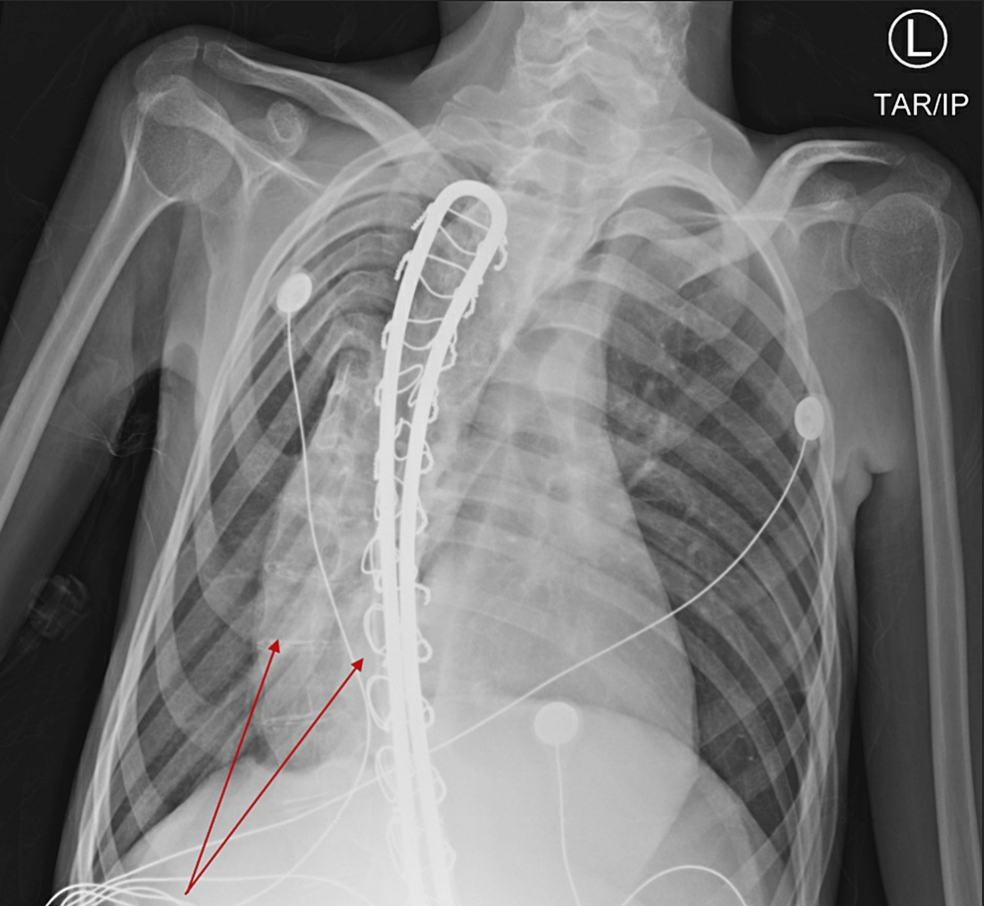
Chest X-Ray Demonstrating Severe Scoliosis and Previous Spinal Fusion (Arrows)
